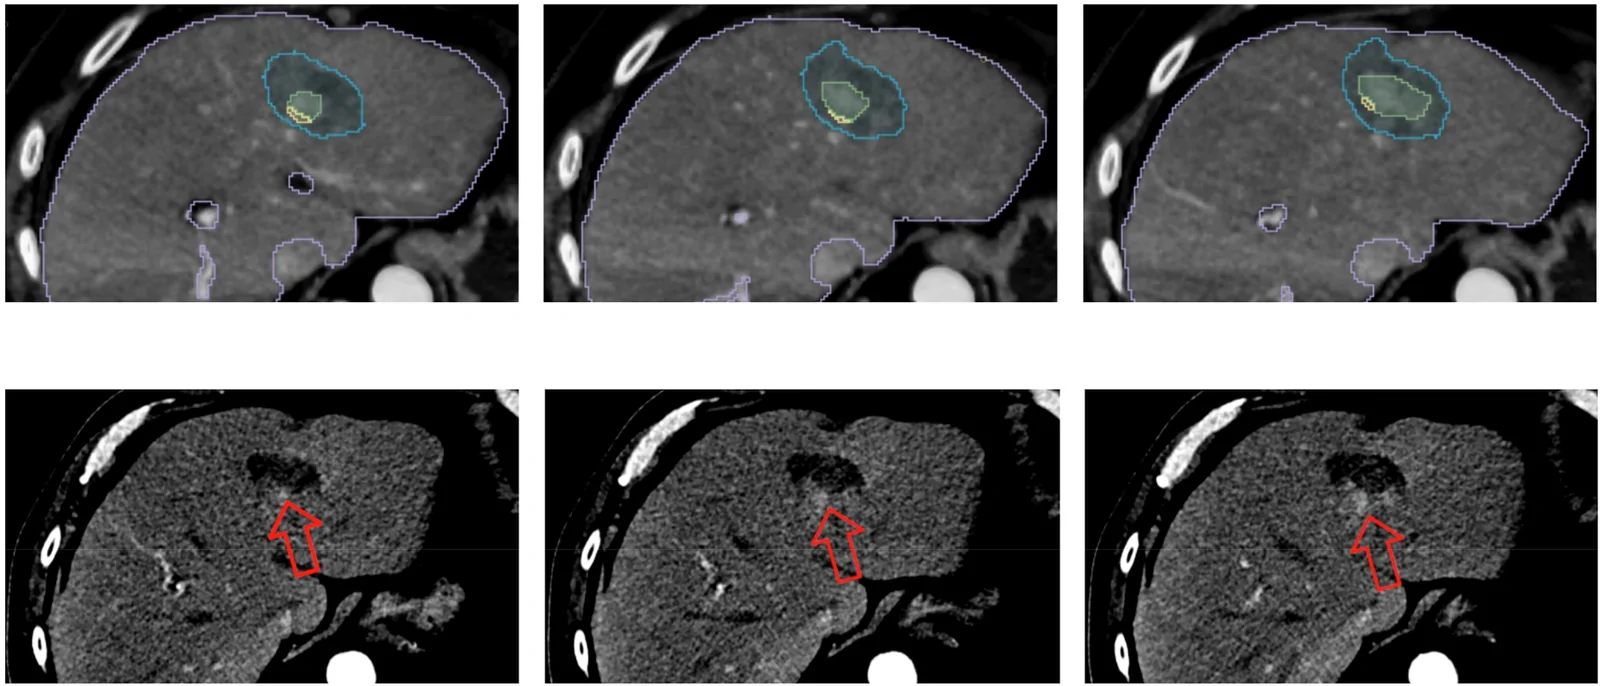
Lesion at risk of developing recurrence (marked in yellow in the top figure), and recurrence visible from the follow-up scan (28 months later). Top row: overlay of pre-interventional arterial CT scan (50%) and coregistered post-interventional portal venous scan (50%) with segmentations and margins (consecutive slices). The liver contour is outlined in purple, while the ablation contour is in blue. Points inside the lesion are colored based on the margin: in green, the points with a sufficient margin, and in yellow, the points at risk of developing recurrence. Bottom row: consecutive slices from the follow-up scan in arterial phase. The location of recurrence is indicated with a red arrow
